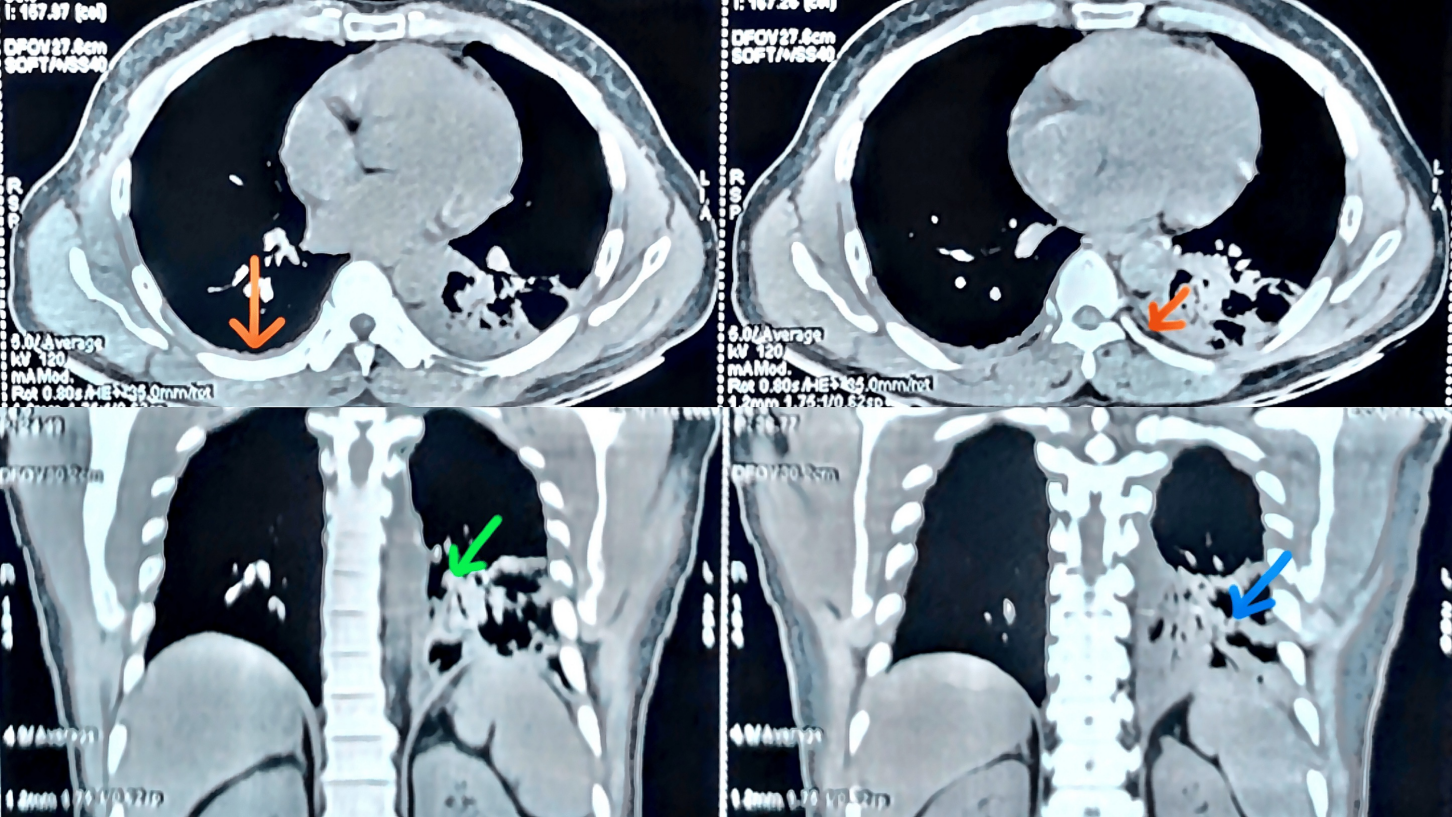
The HRCT thorax image HRCT: high-resolution computed tomography The HRCT thorax demonstrates extensive patchy consolidation in the left lower lobe (blue arrow). Additionally, there is mediastinal and left hilar lymphadenopathy (green arrow), with bilateral minimal posterior basal pleural thickening (orange arrow)
